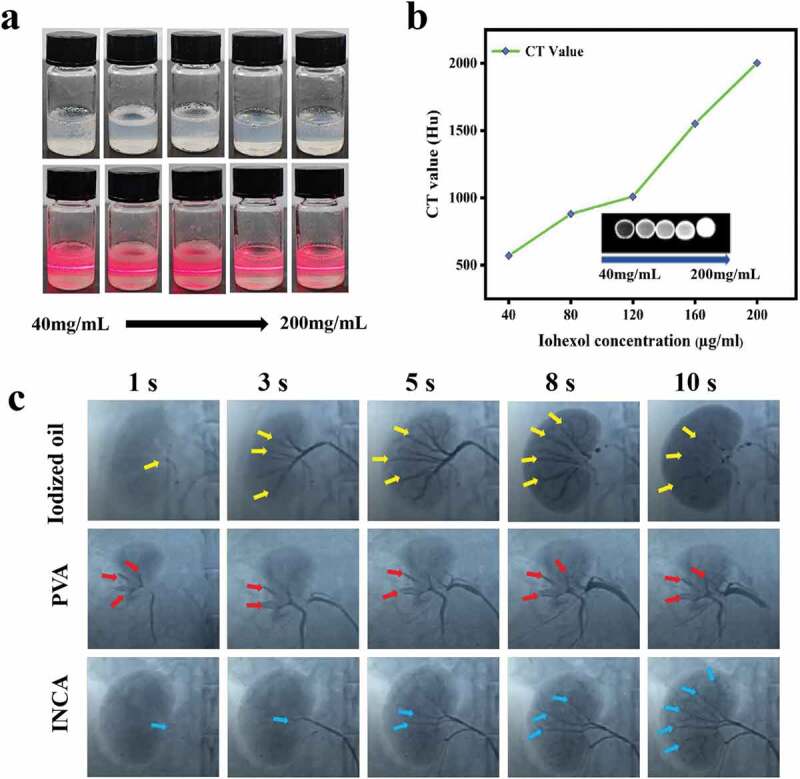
Evaluation of *in-vitro* and *in-vivo* imaging effect of INCA nanogels. (a) Tyndall effect occurred after laser irradiation when NIAPM-*co*-AA nanogels were mixed with iohexol solutions of different concentrations (40 µg/mL, 80 µg/mL, 120 µg/mL, 160 µg/mL, and 200 µg/mL). (b) NIAPM-*co*-AA nanogels were mixed with iohexol solutions containing 40 µg/mL, 80 µg/mL, 120 µg/mL, 160 µg/mL, and 200 µg/mL at different concentrations, and computed tomography was performed to determine INCA nanogels CT values. (c) DSA silhouette of iodized oil, PVA, and INCA nanogels 1s, 3s, 5s, 8s, and 10s after injection into the renal artery.
